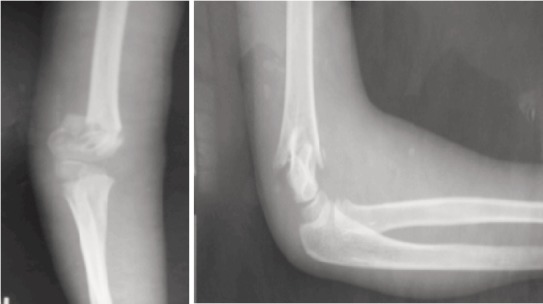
Pre-Reduction X-rays AP and Lateral view of the patient.
